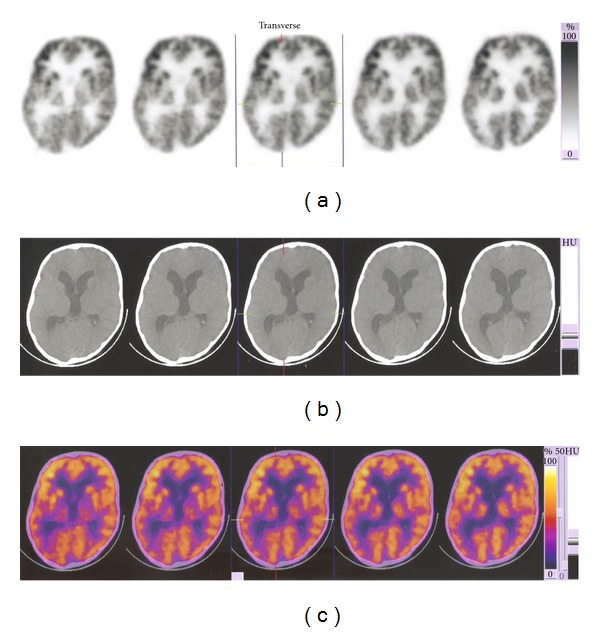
Posttherapy PET and CT scan findings: A slight decrease in the third ventricle dilation and increase in the yellow-orange area in the frontal lobe.
